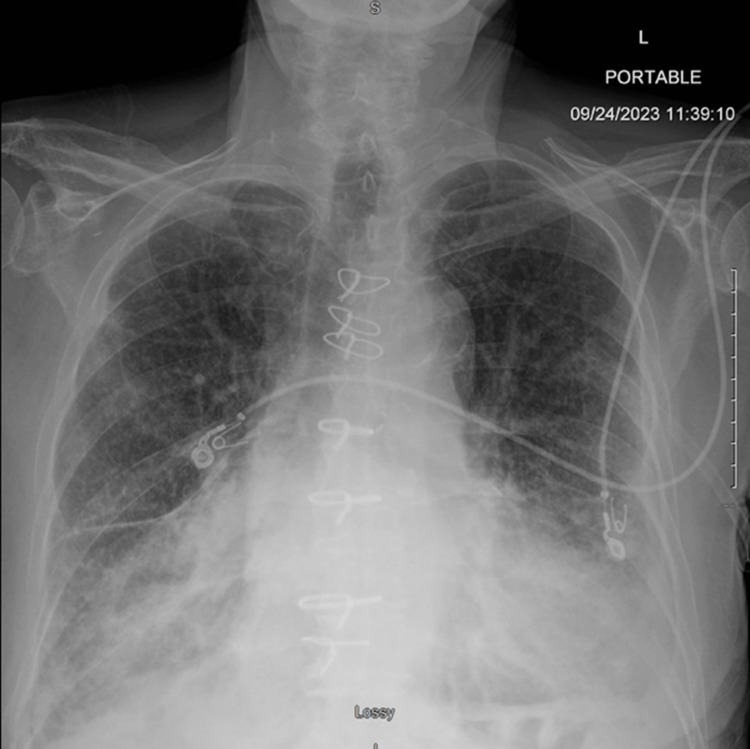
Initial patient chest X-ray. Cardiomegaly with interstitial accentuation which is more prominent in the bases symmetrically. Probable small left greater than right effusions suggestive of congestive failure. This is on a background of probable pulmonary fibrosis.
